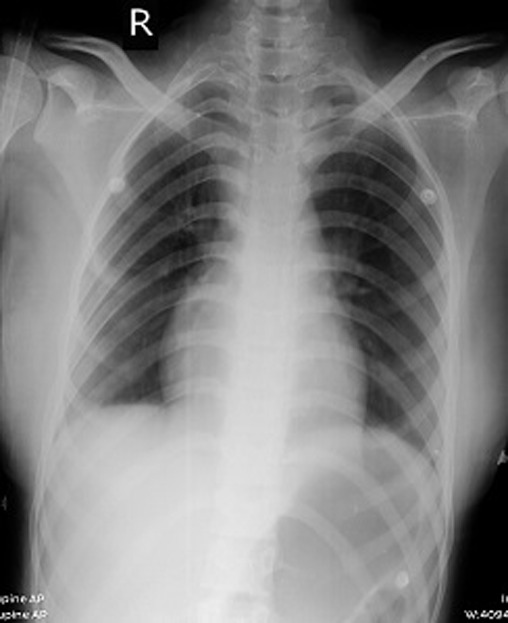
chest X-ray anteroposterior view showing no involvement of lungs
